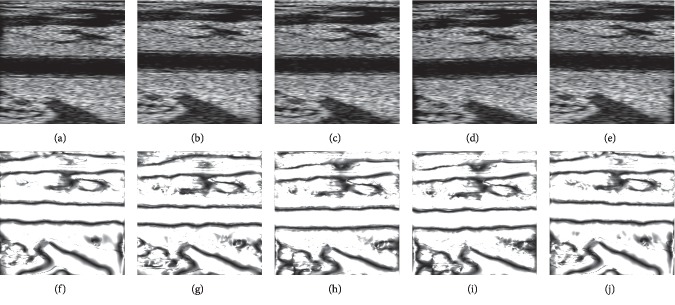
Several frames of the synthetic B-mode ultrasound sequence (a–e) and their corresponding combining phase images (f–j) with wall motion and disturbances at several time points (0 s, 0.9 s, 1.1 s, 2.2 s, and 3.2 s).
